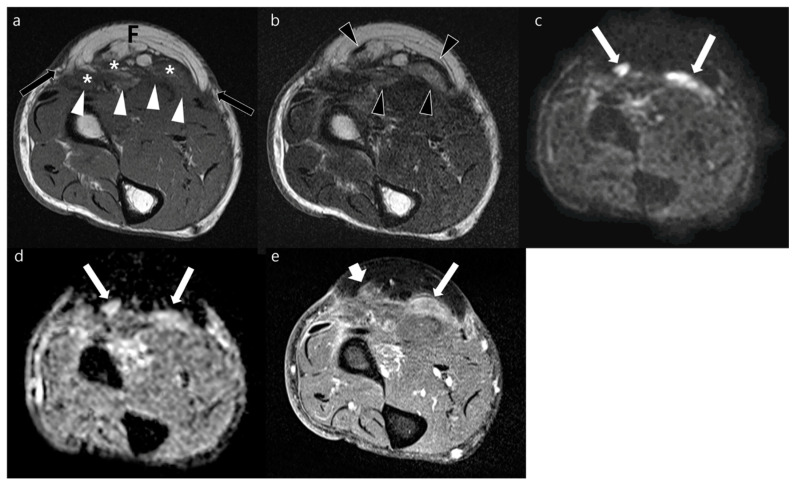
Postoperative MRI obtained from the forearm of a 77-year-old man who underwent surgical resection of myxofibrosarcoma with immediate free flap reconstruction in the forearm 6 months prior. (a) The axial T1WI shows flap (F) with peripheral (arrows) and deep (arrowheads) margins in the volar side of forearm. Ill-defined, heterogeneous lesions (asterisks) are seen along deep margin of the transplanted tissue. (b) Axial T2WI shows ill-defined nodular hyperintense lesions (arrowheads). (c,d) These lesions (arrows) represent facilitated water diffusion seen as hyperintense signal on axial DWI with a *b*-value of 800 s/mm^2^ (c) and high ADC (d). (e) Axial contrast-enhanced FS-T1WI shows focal enhancement of moderate (arrow) and mild (short arrow) degrees. These lesions decreased in size and finally disappeared in enhancement in serial follow-up MRIs without other treatment; they were then presumed as postoperative changes.
